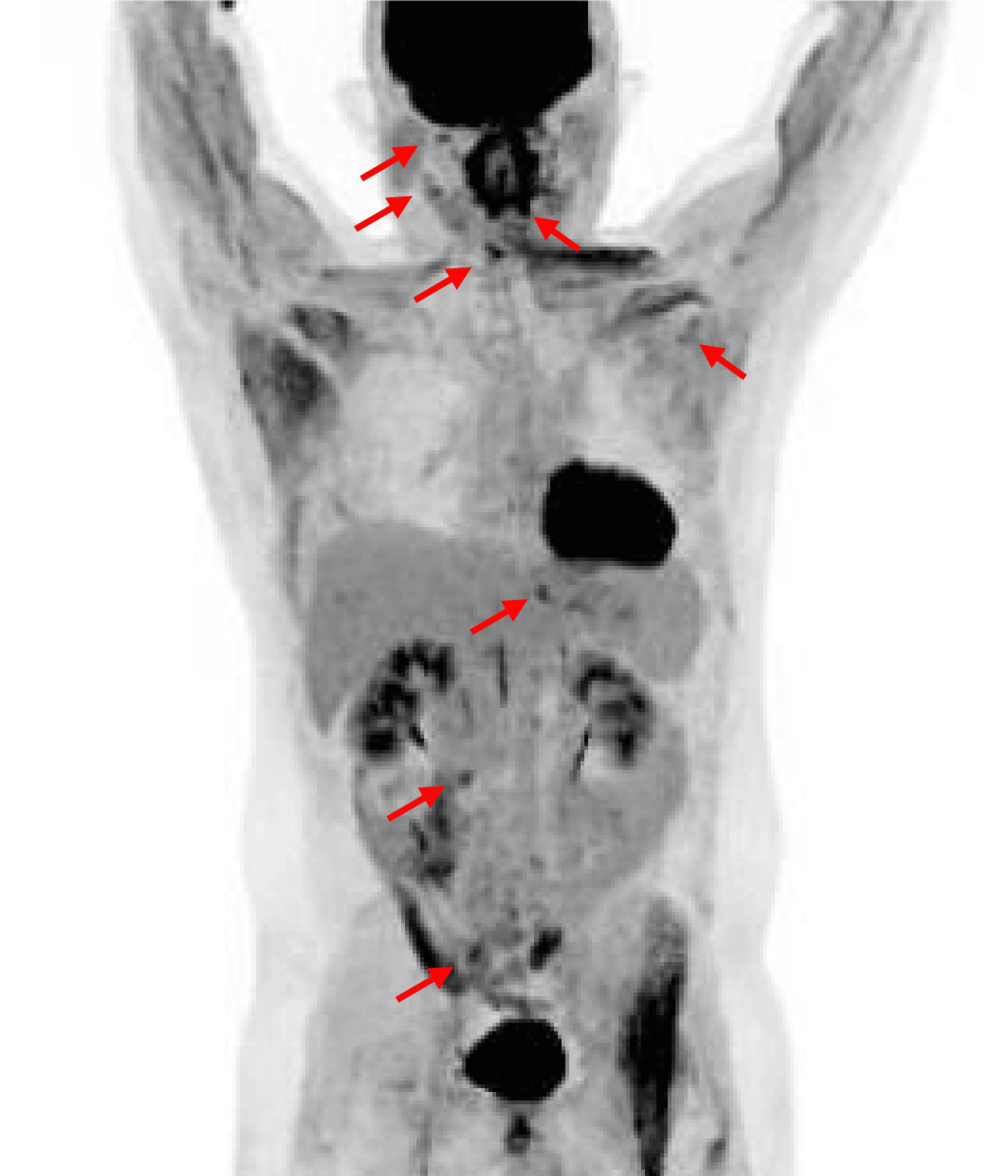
FDG positron emission tomography/computed tomography Mildly increased FDG uptake in the systemic lymph nodes was observed (red arrows). FDG, fluorodeoxyglucose.
